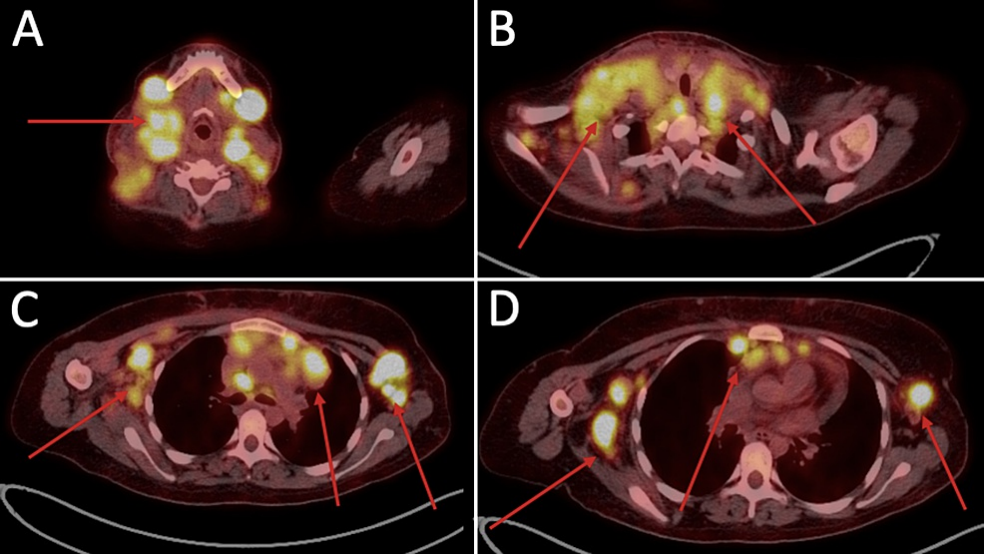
An 18F-FDG PET/CT scan of an 18-year-old female showing lymphadenopathy. A: PET/CT, axial view, representing the bilateral head and neck lymphadenopathy. B: PET/CT, axial view, representing bilateral neck lymphadenopathy. C: PET/CT, axial view, representing axillary and mediastinal lymphadenopathy. D: PET/CT, axial view, representing axillary and mediastinal lymphadenopathy. 18F-FDG PET/CT: positron emission tomography with 2-deoxy-2-[fluorine-18] fluoro-d-glucose integrated with computed tomography; PET/CT: positron emission tomography/computed tomography.
